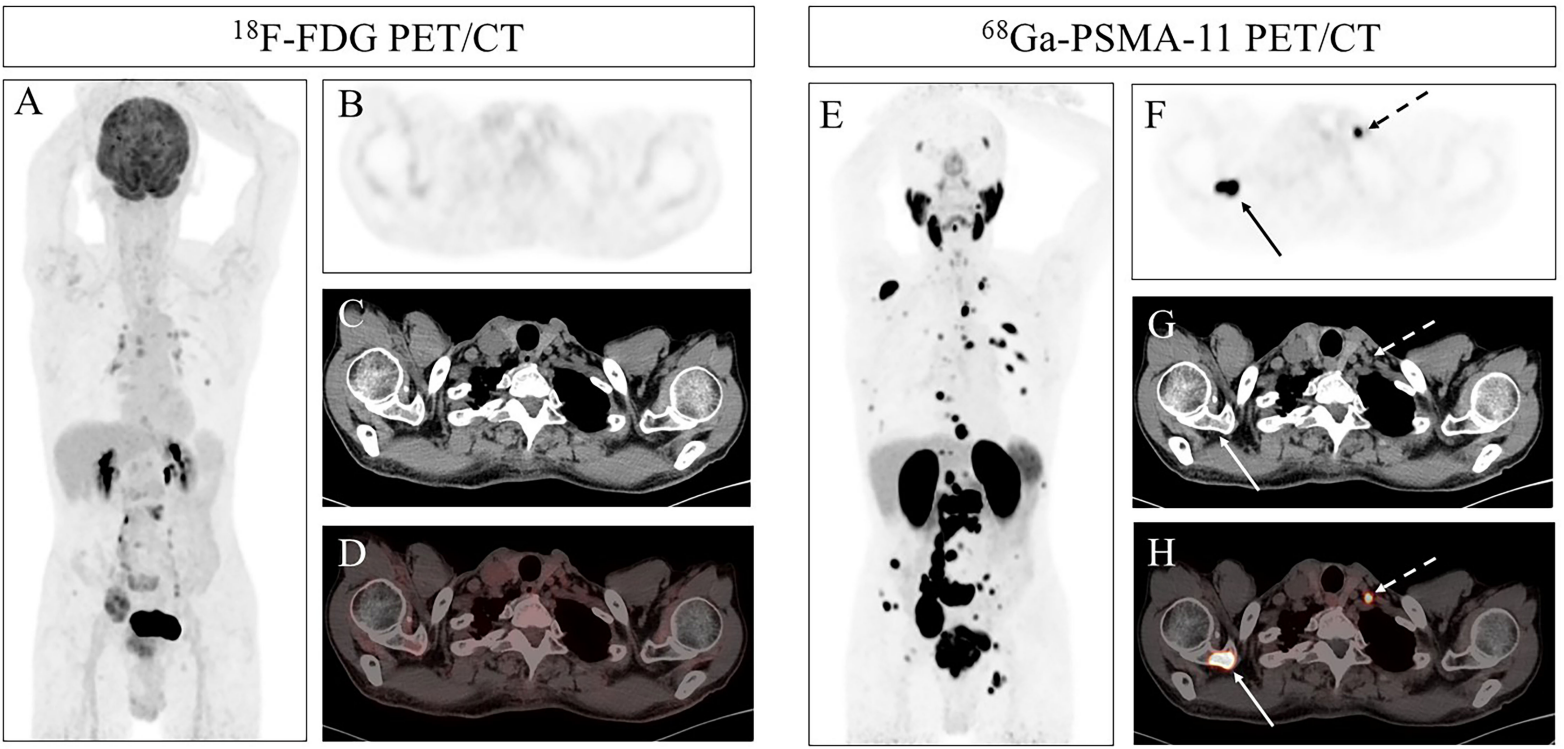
(Patient 14) A 69-year-old man presented with a 2-year history of weak stream and 2-month history of lower-back pain. Pelvic MRI suggested prostate cancer. ^18^F-FDG PET/CT Maximum-intensity-projection (MIP) and axial images (A, B) showed no hypermetabolic foci in the left supraclavicular region. Concomitant CT and fused PET/CT slices (C, D) confirmed absent FDG uptake in this area. ^68^Ga-PSMA-11 PET/CT MIP and axial views (E, F) revealed intense PSMA expression in a left supraclavicular lymph node (dashed arrow; SUVmax 14.81) and in the right scapula (solid arrow; SUVmax 41.39). Furthermore, ^68^Ga-PSMA-11 PET/CT MIP showed additional bone metastasis lesions compared to ^18^F-FDG PET/CT. CT and fused images (G, H) precisely localized both lesions, which were subsequently verified as metastatic on follow-up imaging.
